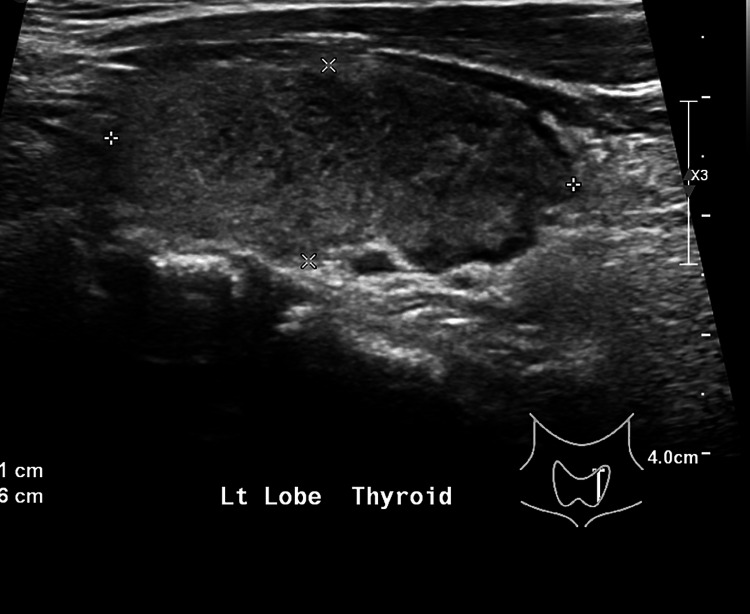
Thyroid US showed a mildly enlarged thyroid (left lobe) with diffusely heterogeneous echogenicity, suggestive of thyroiditis. US: ultrasonography.
